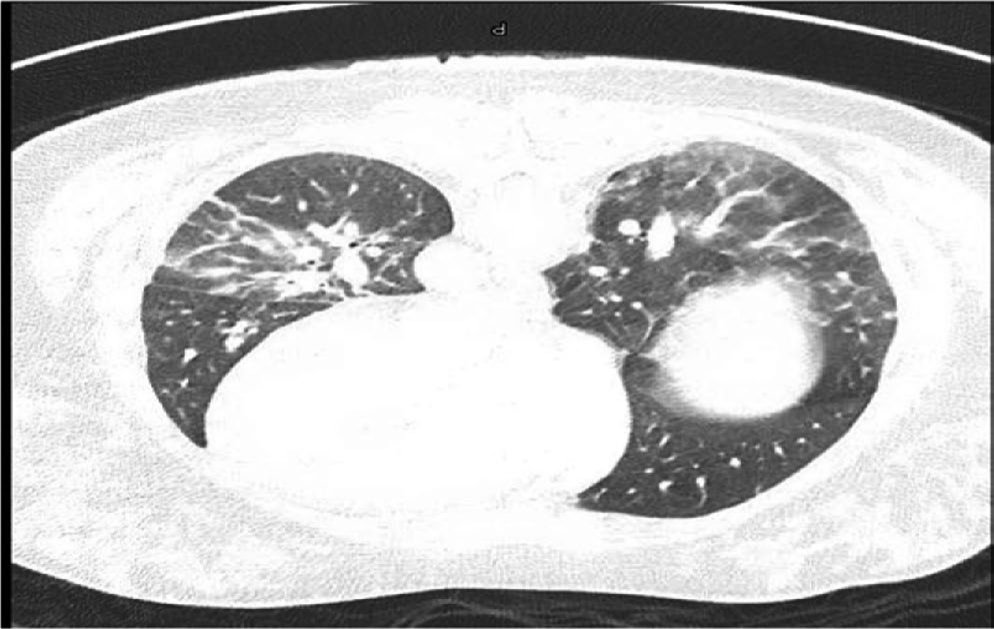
High‐resolution CT pulmonary angiogram showed no evidence of pulmonary embolism but bilateral ground glass opacities
